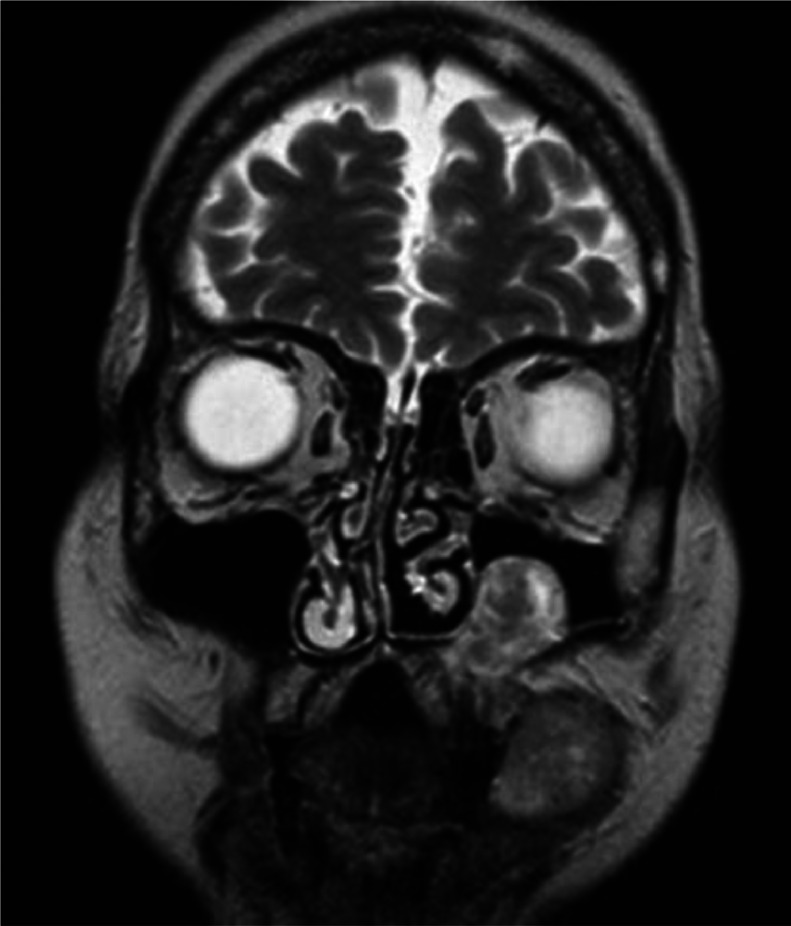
MRI T2 TSE coronal plane. The lesion showed mild T2 signal hyperintensity with erosion of the left alveolar process and homolateral maxillary sinus. Erosion extended inferiorly to the hard palate.
